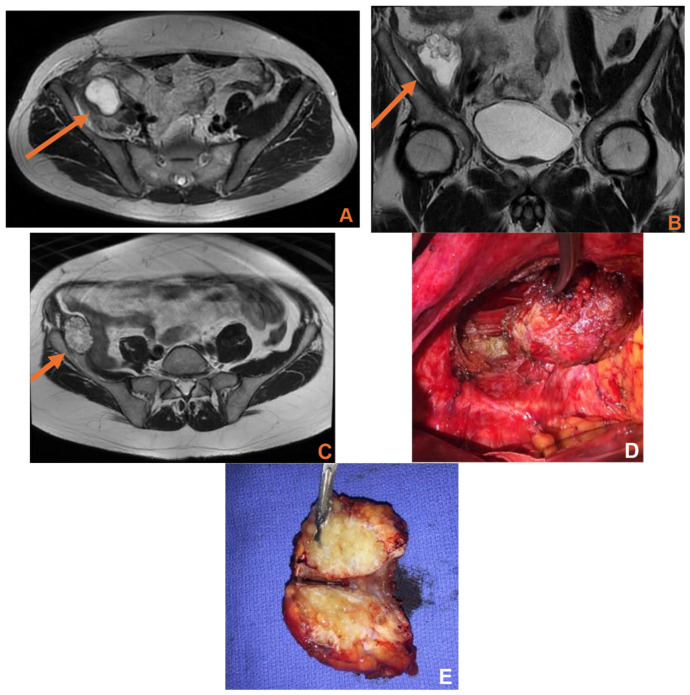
Appendiceal adenocarcinoma. (A–C) MRI demonstrating a fluid collection in the right iliac fossa associated with a tumoral mass and invasion of the iliopsoas muscle (arrow). (D,E) Intraoperative view of the local recurrence, which was completely resected.
